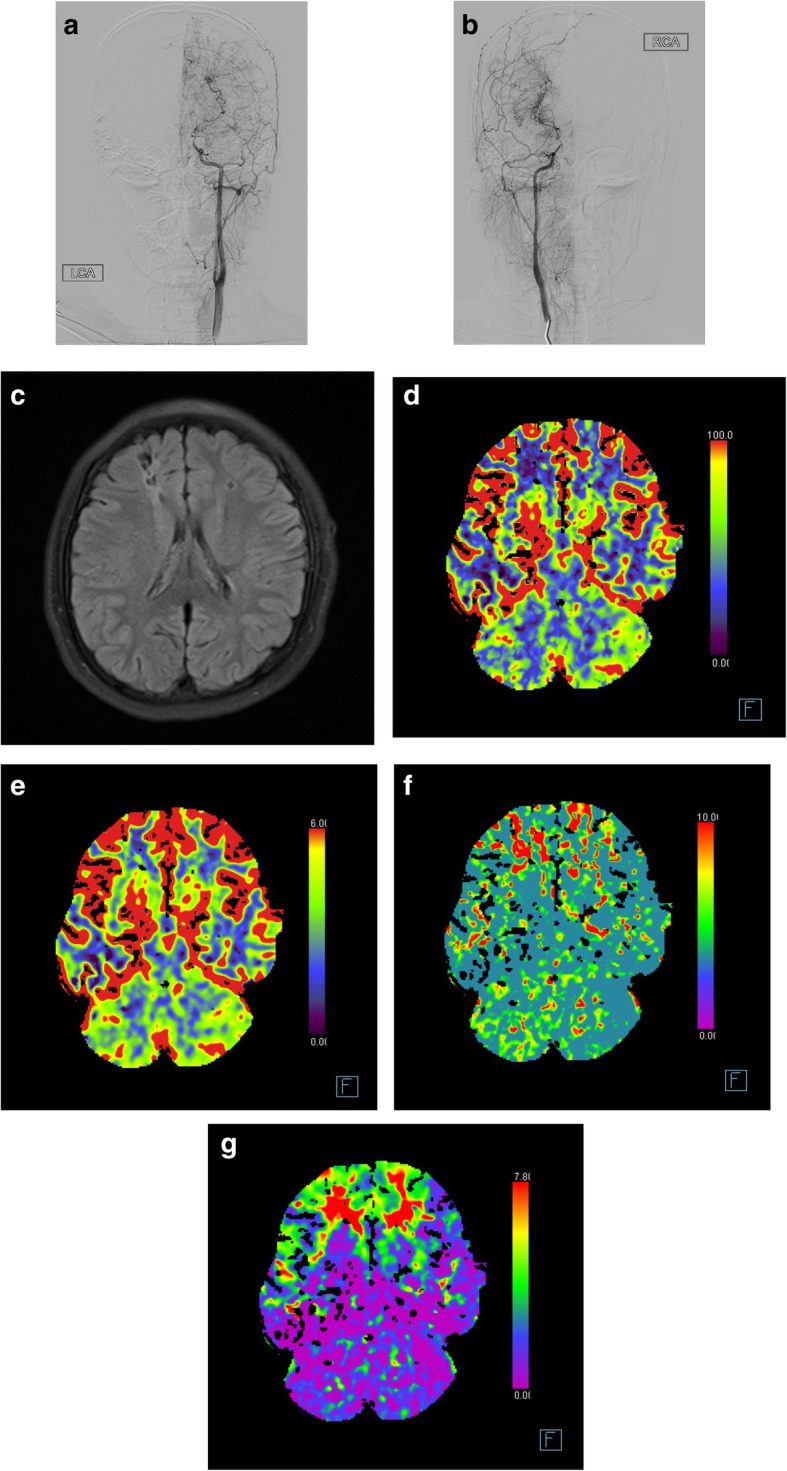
Clinical data of a 9-year-old girl. a and b Bilateral severe stenosis of terminal internal carotid artery on DSA (a left; b right). c Infarction in both frontal lobes on MRI. d–g Hypoperfusion in both frontal lobes on CTP. Reduced cerebral blood flow (d), relatively normal cerebral blood volume (e), increased mean transit time (f), and increased Tmax in both frontal lobes (g)
